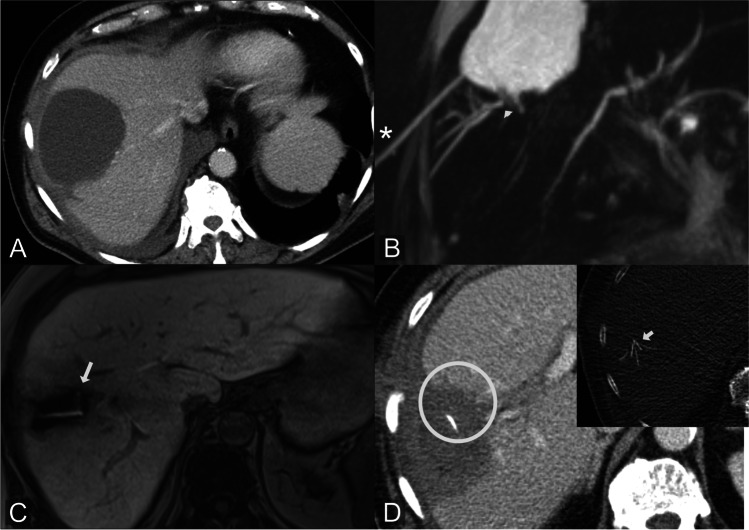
Disconnected duct: CT of a 71-year-old patient with colorectal liver metastases after surgical enucleation and postoperative biloma (A). T2w-MRCP demonstrates the biloma after percutaneous drainage (asterisk) and displays a disconnected segmental bile duct opening into the biloma (arrowhead) (B). Gd-EOB-DTPA-MRCP proves peripheral BL from this disconnected duct into the biloma at the resection site (C). Leakage was subsequently treated by radiofrequency ablation of the disconnected duct. CT fluoroscopy of probe placement (short arrow) and the corresponding ablation zone in post-interventional contrast-enhanced CT are shown (D)
